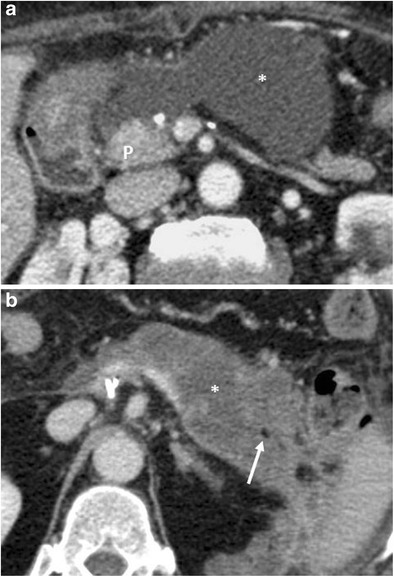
Postoperative collections (a, b). a Axial CT image shows a homogeneous fluid collection with a thin wall at the level of the resection margin and in the surgical bed (*) after a distal pancreatectomy. The head of the pancreas (p) is visualised. b An inhomogeneous necrotic fluid collection (*) with fat globules inside (white arrow) is visible within the left anterior pararenal fascia. These findings are consistent with a necrotic collection with steatonecrosis due to a postoperative pancreatitis after a Whipple procedure
